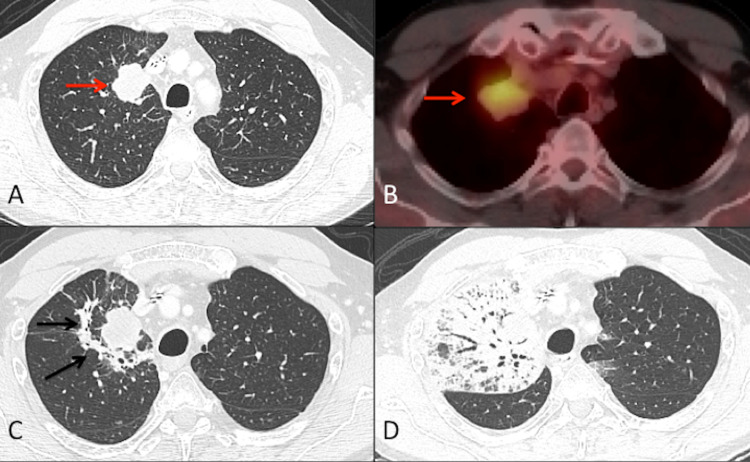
Pre- and post-irradiation imaging Axial section CT chest lung window (A) and axial section fused FDG PET/CT image (B) show lung mass in the upper lobe of the right lung with hypermetabolic uptake of FDG (red arrow) suggesting lung cancer. Axial section of follow-up CT chest (C) after five months of radiation therapy shows early post-radiation fibrosis surrounding the treated mass (black arrows). Follow-up CT chest (D) on March 18, 2021, at eight months after the completion of radiation therapy and after the administration of the second COVID-19 vaccine. The image shows extensive consolidation with surrounding ground-glass opacities and bronchiectasis within the prior radiation therapy portal suggestive of “Radiation Recall” type of pneumonitis (corresponding coronal image of Figure 2D within Figure 3) CT: computed tomography; PET: positron emission tomography; FDG: fluorodeoxyglucose; COVID-19: coronavirus disease 2019
